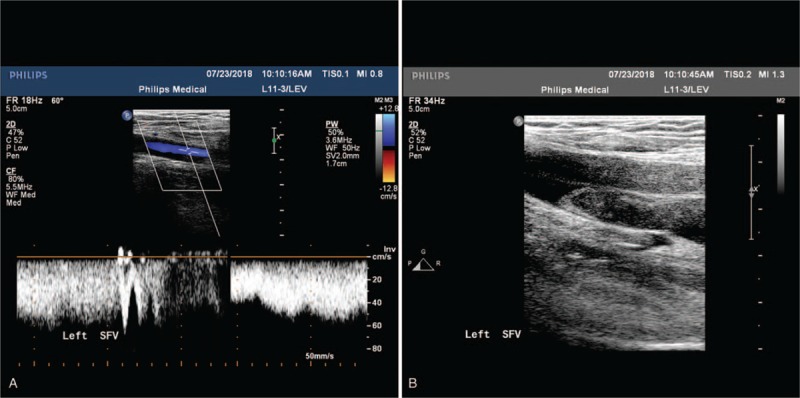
Infrainguinal venous Duplex ultrasonography findings. (A) Valvular reflux in the left superficial femoral vein (SFV). (B) Venous thrombus in the left SFV.
